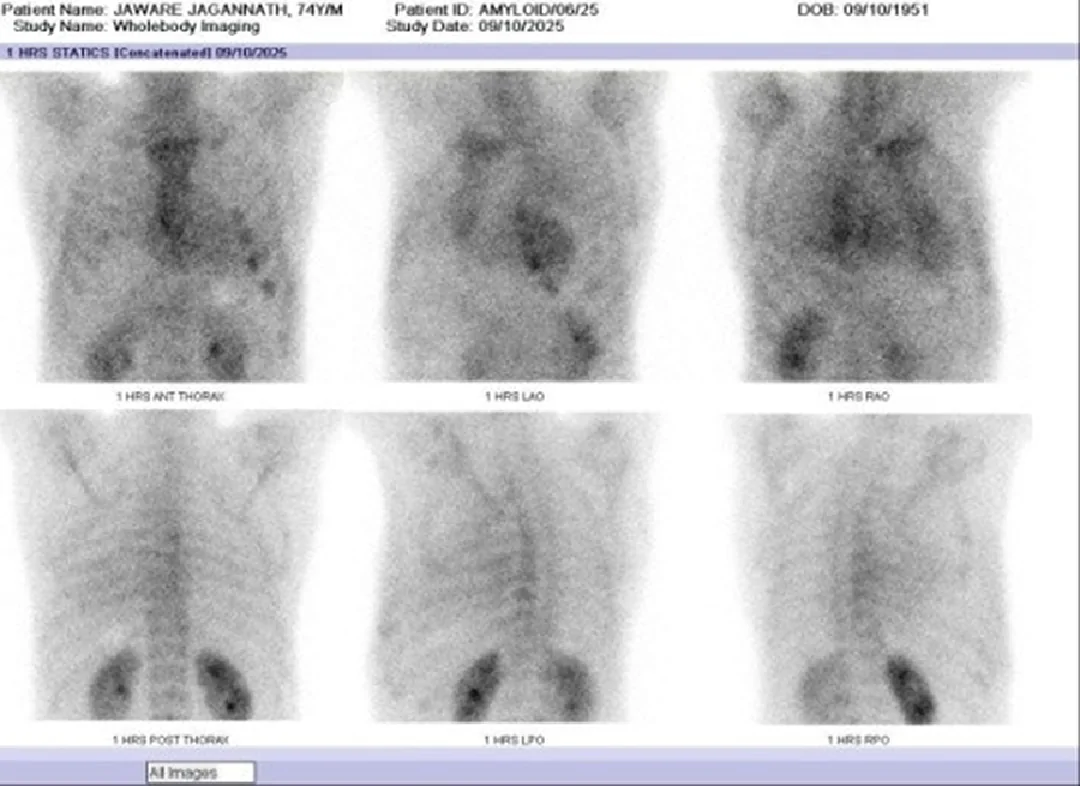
Planar 99mTc-pyrophosphate (99mTc-PYP) scintigraphy performed 1 h after tracer administration. Anterior, left anterior oblique (LAO), right anterior oblique (RAO), posterior, left posterior oblique (LPO), and right posterior oblique (RPO) thoracic projections demonstrate diffuse, intense myocardial tracer uptake exceeding adjacent rib uptake (Perugini grade 3), highly suggestive of transthyretin cardiac amyloidosis.
